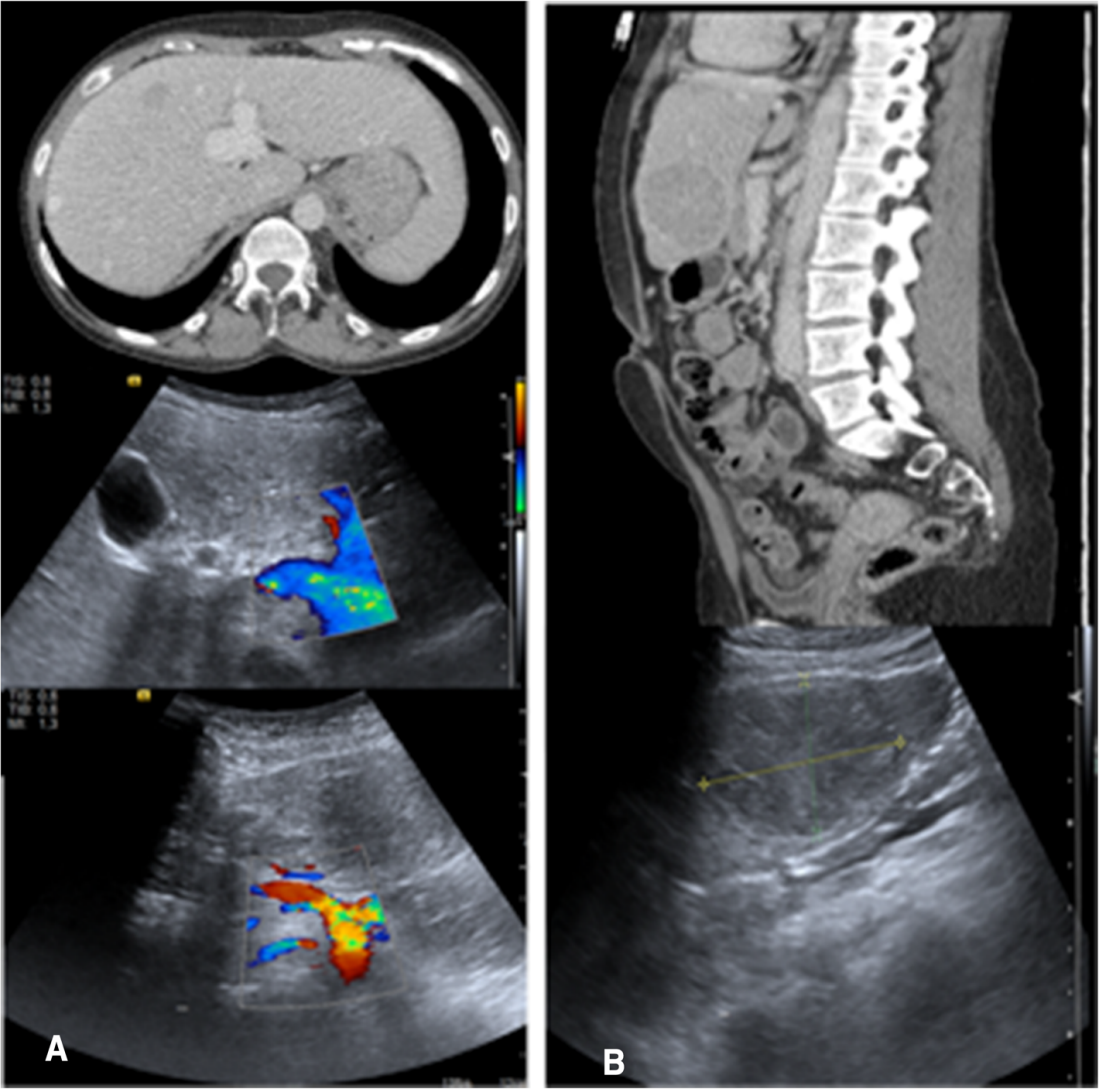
Correlation between CT and Color Doppler Duplex Ultrasound in axial and sagittal planes. Note: Case 1 (Type 1) a: Axial CT plane in the venous ligament level with left branch of portal vein extra hepatic dilated. Color Doppler Duplex US below shows us an extra hepatic portal route and a dilated hepatic artery emerging from the celiac trunk. b: Sagittal planes show a hypodense lesion in segment III (50 X40 mm) and predominantly hypoechoic, respectively at TC and the US
